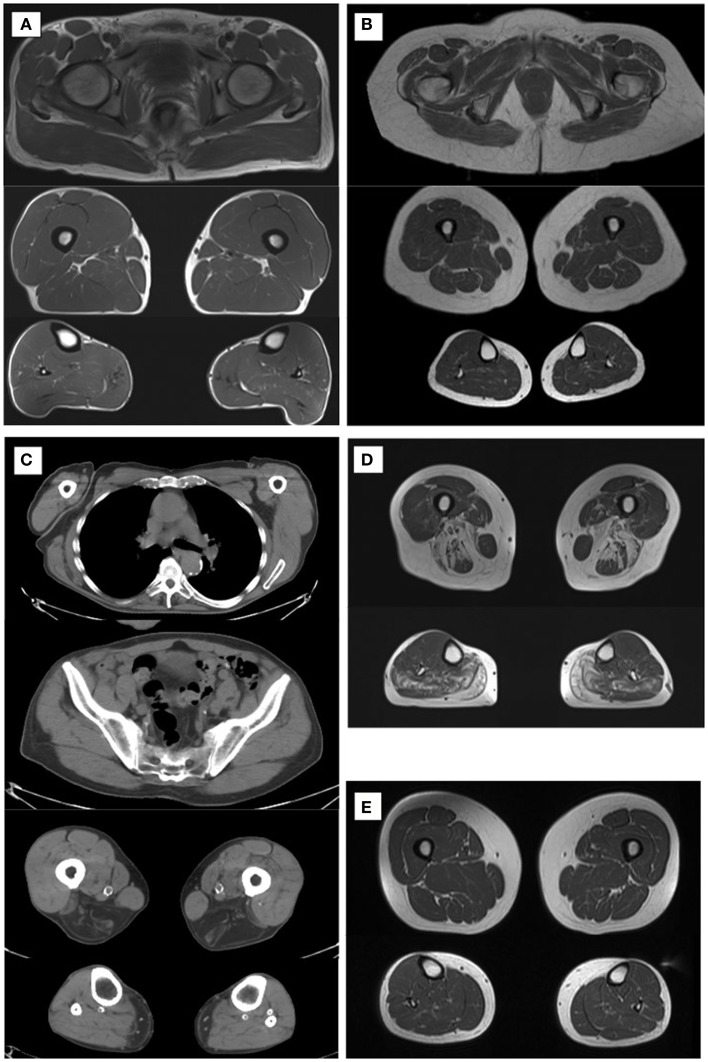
Muscle imaging at different ages and diseases. Normal muscle imaging in a 40-year-old male (A). Normal muscle imaging in a 73-year-old male with clear decrease in muscle volume (B). Slight increase of diffuse fatty tissue is seen intramuscularly. Muscle CT in a 78-year-old male with LGMD2L shows dystrophic changes in the hamstrings and gastrocnemius medialis muscles (C). Muscle MRI findings in a patient with LGMD1D at age 73 with typical fatty degenerative changes in the hamstring muscles and adductors at thigh level in the soleus and gastrocnemius muscles in the calves (D). Muscle MRI in a patient with DM2 (E) shows that muscle volume is normal, and fatty degenerative changes are not seen at age 60.
